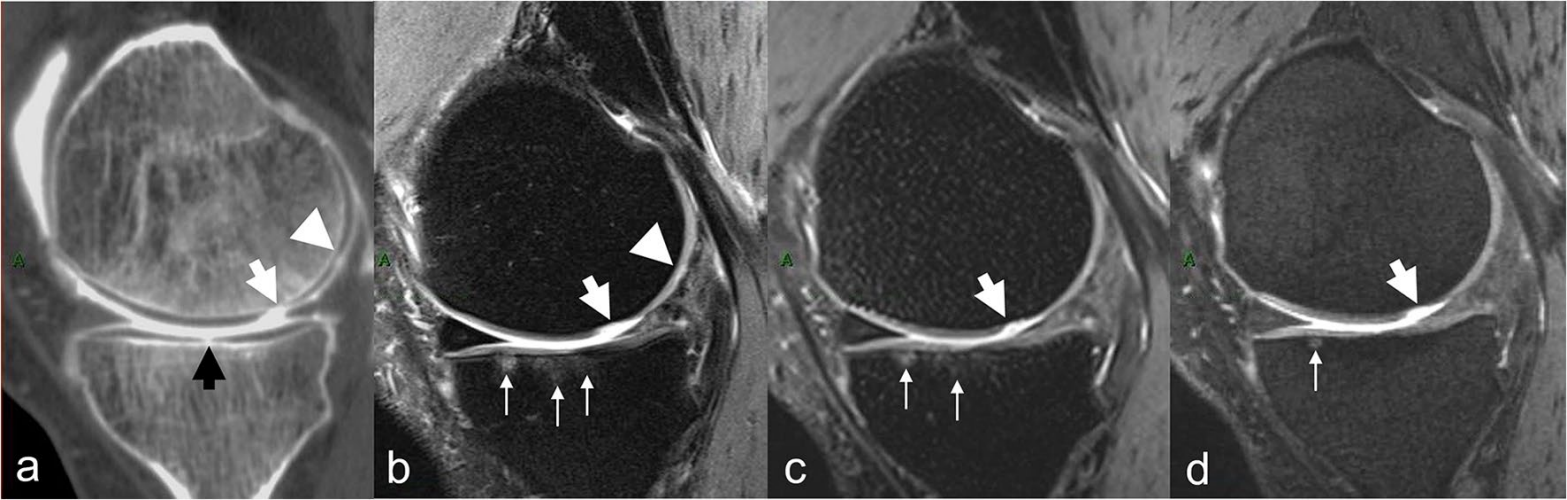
a Sagittal reformat of a CT arthrogram of the knee and corresponding b sagittal 2D fast spin-echo (FSE) fast-suppressed (FS) intermediate-weighted (IW), c sagittal reformat of 3D FSE IW FS and d sagittal reformat of 3D dual echo steady state (DESS) MR images obtained on a 3T MRI unit on the same day. Area of deep cartilage substance loss (arrow in a) is visualized on all MR images, whereas the superficial cartilage substance loss is only well depicted on 2D IW FS (arrowhead in a and b). Subchondral bone marrow edema-like signal intensity is visualized on 2D and partially on 3D IW FS (thin arrows in b and c) but much less conspicuous on 3D DESS image (thin arrow in d)
